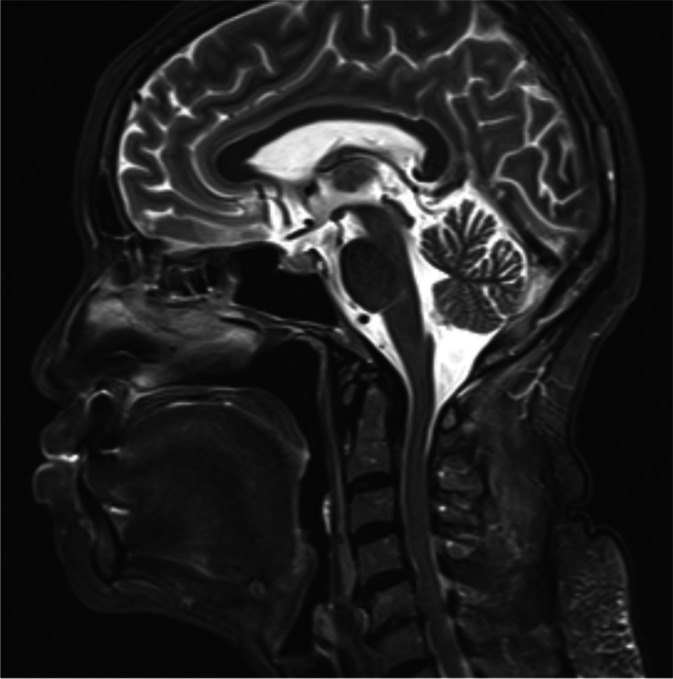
Sagittal view of the head showing macroglossia. T2-weighted magnetic resonance imaging revealed the enlarged tongue. Swelling at the base of the tongue was mild with no airway obstruction
